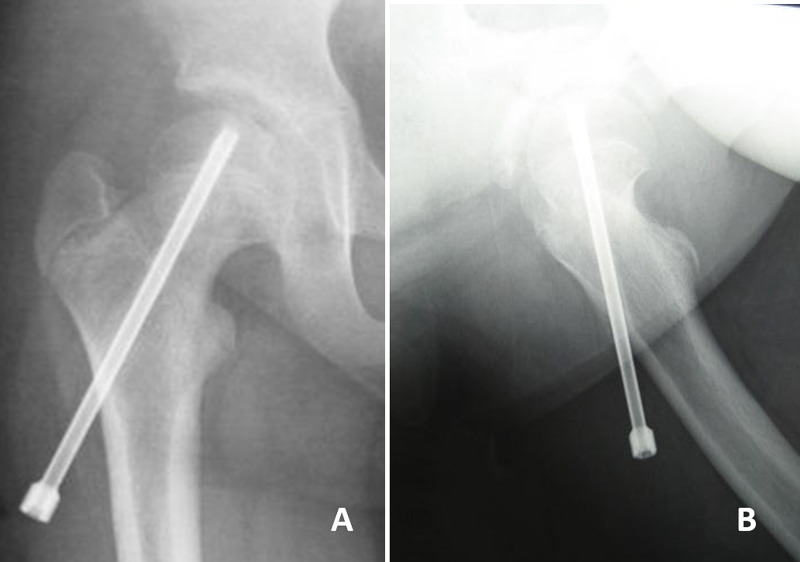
Postoperative imaging (second operation; 3 years 4 months after first surgery). (A) Anteroposterior and (B) Rauenstein radiographs of the right hip; we replaced the other longer screw through the same screw hole.
